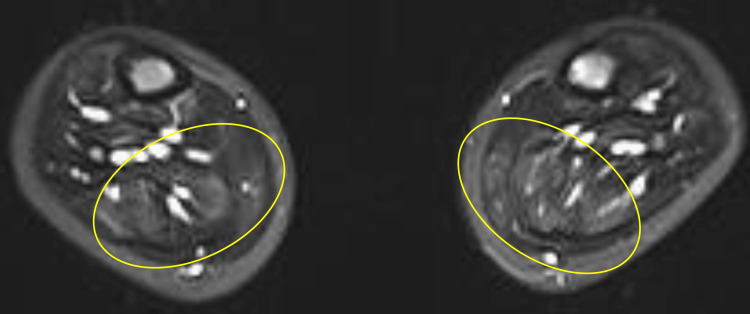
The transverse section of the MRI scan using STIR shows multiple high-signal intensities, predominantly in the lower limbs. The yellow rings indicate areas of signal change STIR: Short tau inversion recovery
